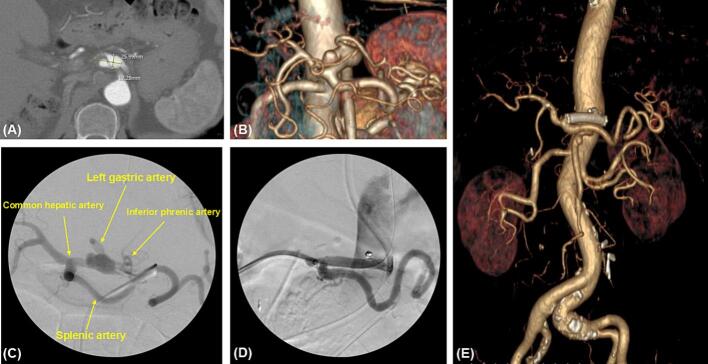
Preoperative, intraoperative, and postoperative images of the patient. (A) The preoperative axial view of the computed tomography scan showed that the size was approximately 26 mm in width and 12 mm in height. (B) The volume-rendering image of preoperative computed tomography showed the saccular shaped pseudoaneurysm in the celiac artery. (C) Intraoperative contrast angiogram showed the aneurysm of celiac artery on the first operation. (D) Completion angiogram after hybrid surgery showed the complete resolution of aneurysm and no endoleak. (E) Postoperative volume-rendering view confirmed that the aneurysm was we completely excluded without an endoleak, and the flow of the common hepatic artery and splenic artery showed good patency.
